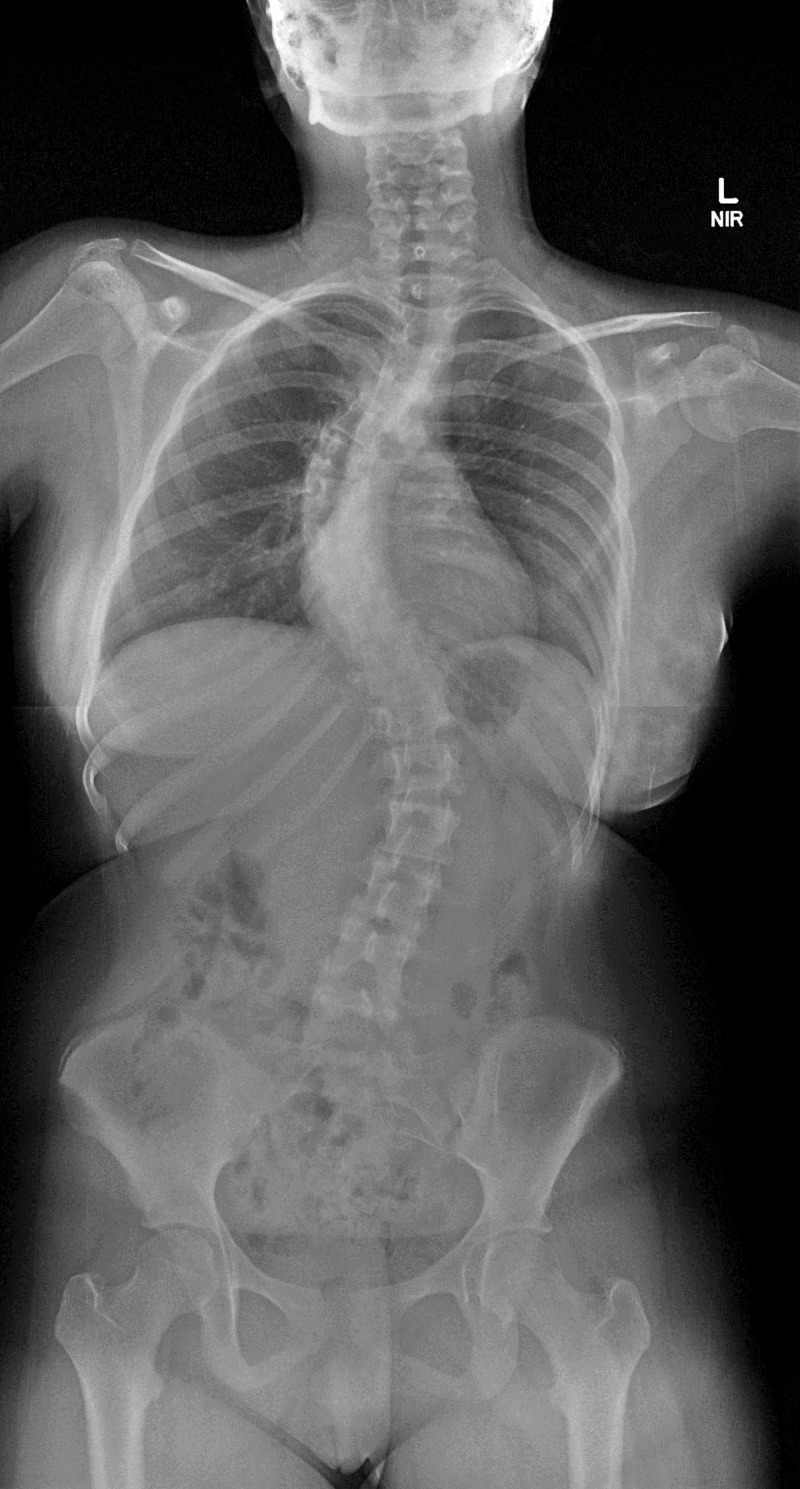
Preoperative AP radiograph demonstrating the degree of scoliosis curvature 53 degree right convex thoracic curve from T4-T10 and 49 degree left convex thoracolumbar curve from T11-L3 AP: anteroposterior
